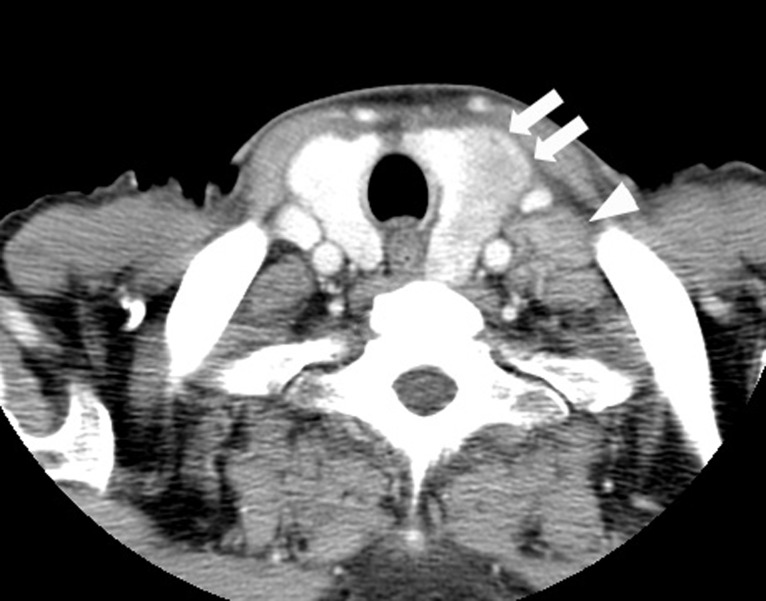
CT findings of the thyroid tumor. Thyroid tumor in the left lobe (arrows) and the left cervical lymph node swelling (arrowhead) are detected in contrast-enhanced CT. This thyroid tumor is a heterogeneously low-density tumor with an unclear border. The trachea is deviated to the right by this tumor.
